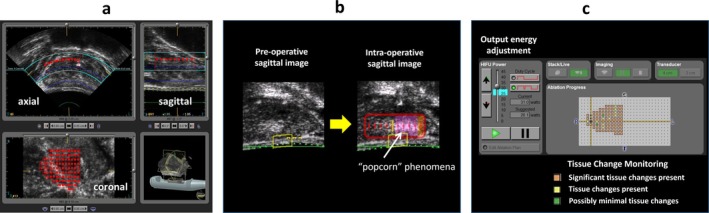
Treatment planning and real‐time monitoring in HIFU. (a) Cross‐sectional treatment planning: High‐intensity focused ultrasound (HIFU) treatment planning is performed using three cross‐sectional views of the prostate: Axial, sagittal, and coronal. These views enable precise localization of the treatment area, ensuring accurate energy delivery to the targeted region. (b) The “popcorn” phenomenon refers to the appearance of hyperechoic areas within the treated region on B‐mode ultrasound imaging during HIFU therapy. This phenomenon is caused by tissue cavitation during treatment and serves as an indicator that sufficient energy has been delivered to the targeted area, confirming that effective ablation has been achieved. (c) Real‐time energy adjustment and tissue monitoring: The Sonablate device allows for real‐time TRUS imaging, enabling energy adjustments based on the appearance of cavitation phenomena and continuous tissue change monitoring, ensuring optimal treatment precision.
